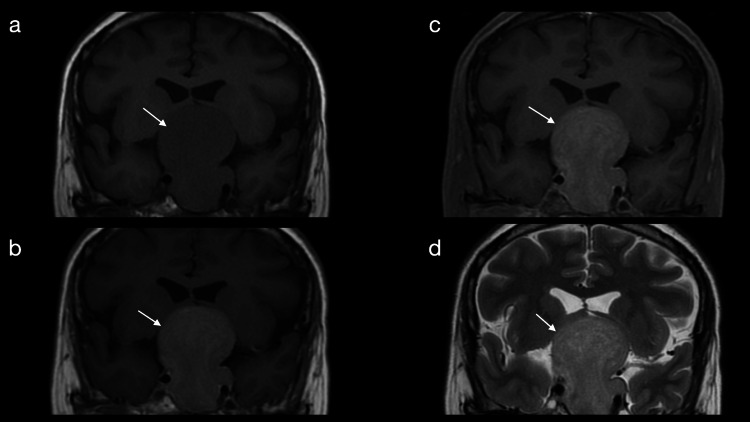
An MRI of the skull with a non-functional pituitary macroadenoma is observed in coronal cuts in sequences (a) simple T1, (b) contrasted T1, (c) T1 contrasted with fat suppression, and (d) T2. MRI: magnetic resonance imaging.
